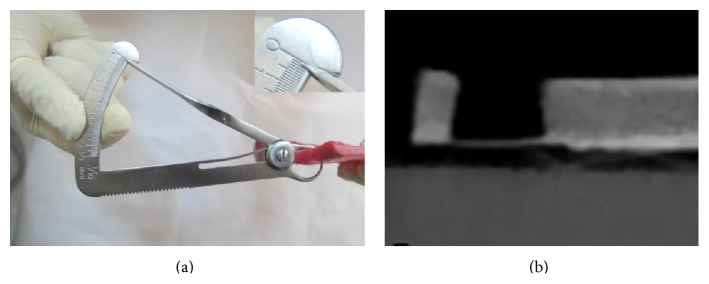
(a) The remaining cortical bone was measured by dental caliper (here in sample number 1 the thickness is 0.1 mm). (b) In cross-sectional view of CBCT image, no perforation is found.
